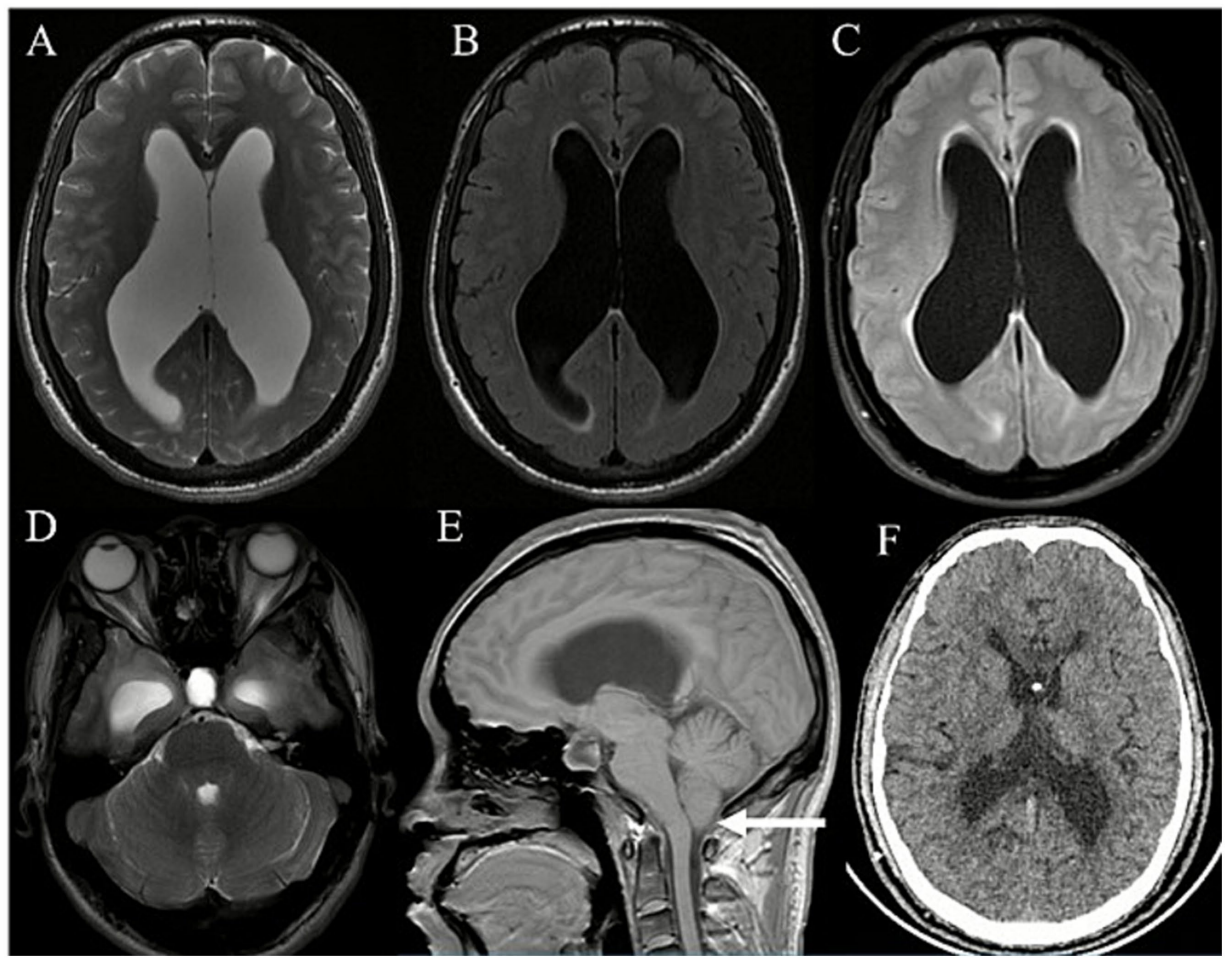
Prior to MVD, brain MRI revealed bilateral ventricular enlargement (A,B) with mild interstitial brain edema (B). Post-MVD and pre-VPS brain MRI revealed persistent bilateral ventricular enlargement, exacerbated interstitial brain edema (C), and a normal-sized fourth ventricle (D). In addition, there was evidence of cerebellar tonsillar herniation [(E); white arrow]. Post-VPS brain CT indicated a reduction in ventricular size (F).
